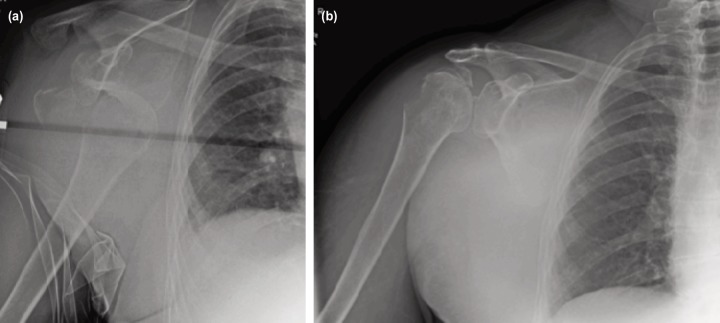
Radiographs of right shoulder (a) AP view showing anterior fracture dislocation, (b) Post-manipulation showing reduction of dislocation and displaced greater tuberosity fracture fragments.
